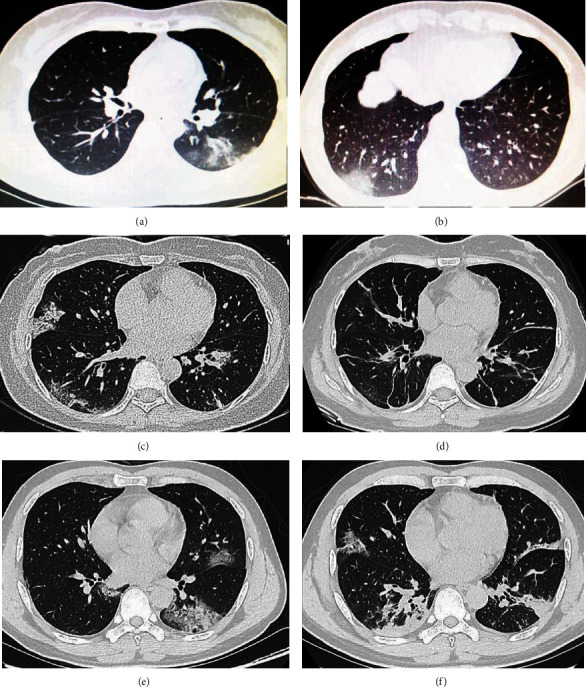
Chest computed tomographic images of three cases infected with COVID-19. (a, b) Computed tomography images of case A obtained on the onset of illness and the admission, respectively. (c, d) Computed tomography images of case B taken before and after the treatment, respectively. (e, f) Computed tomography images of case C indicated the development of the pneumonia.
